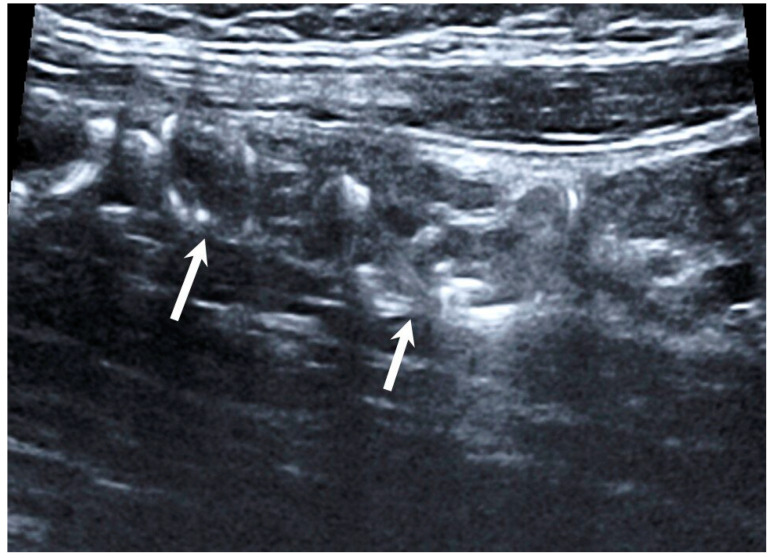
Duplex ultrasound scanning. Hyperechoic structures (coils) in the lumen of the gonadal vein (arrows).
